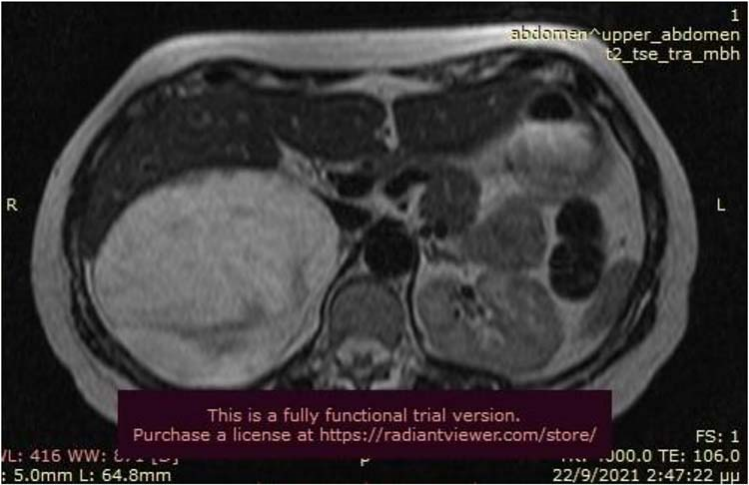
MRI scan depicted a 16 × 15 × 6 cm right adrenal mass with characteristics suggestive of a myelolipoma (transverse plane).
